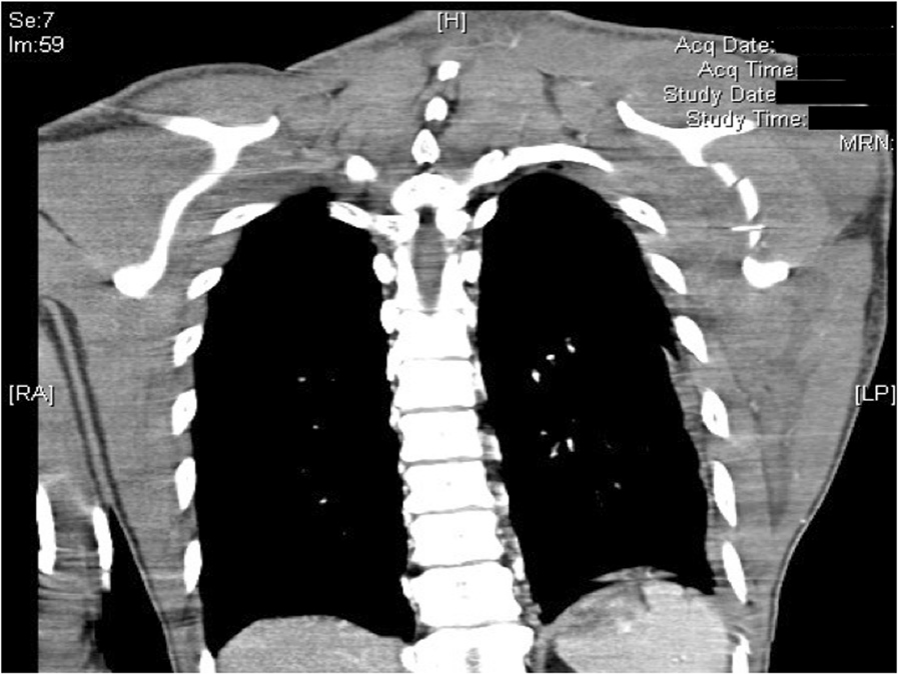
CT thorax coronal view. CT thorax showing comminuted fracture of the left scapula body and left chest wall soft tissue swelling
